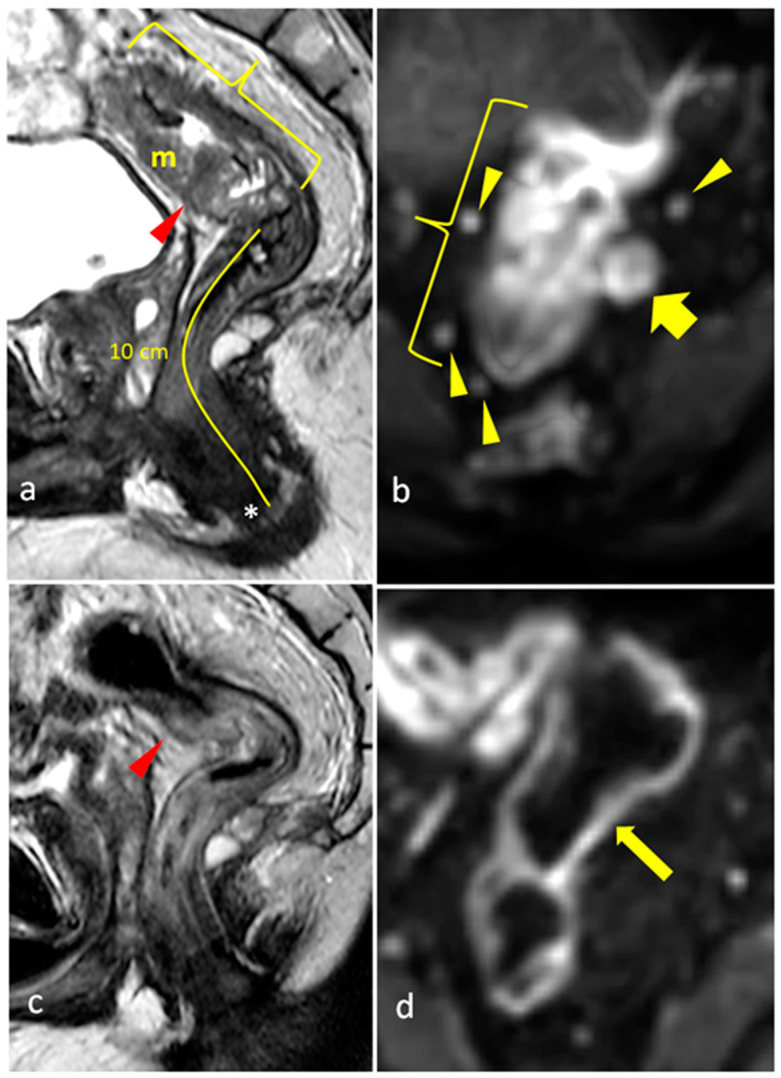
Pretreatment (a) sagittal T2-weighted TSE showing intermediate signal intensity and high rectal mass (m), seen at 10 cm from the anal verge (*) extending for a craniocaudal distance of 8 cm (bracket) and encroaching on the peritoneal reflection (red arrowhead). (b) Coronal DWI of the same mass showing diffusion restriction, associated with several mesorectal lymph nodes (arrowheads) and a mesorectal metastatic deposit (short arrow). Post-treatment (c) sagittal T2-weighted TSE showing complete radiologic response with rectal wall edema at the site of the mass (thickened rectal wall with high signal intensity), and corresponding (d) DWI showing resolution of the mass and metastatic deposit (mrTRG 1).
